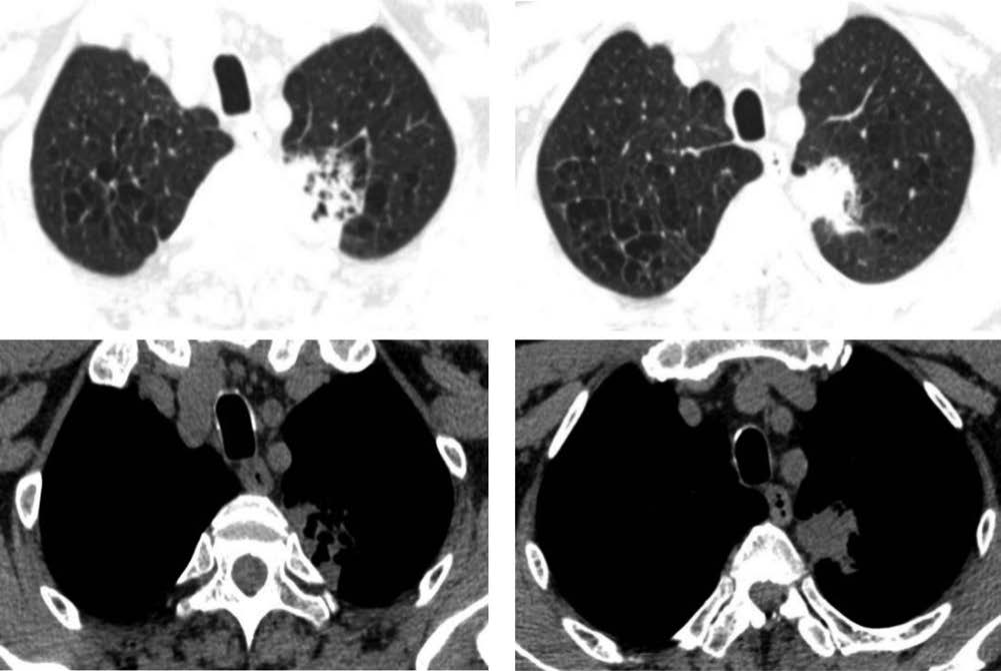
The result of a chest CT of the patient in our hospital on May 19, 2021.
